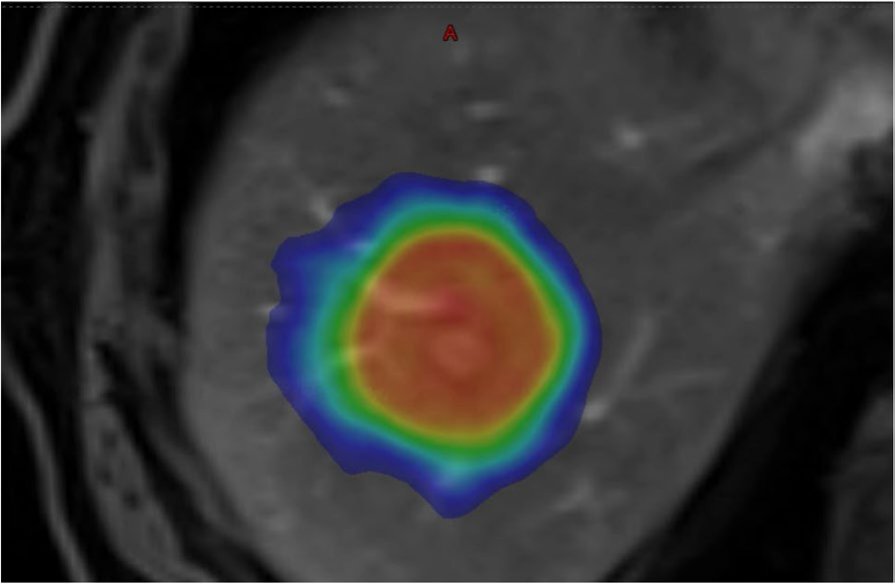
Planning MRI image of SBRT (24Gy / 3#) dose wash to a liver metastasis (image T1 weighted, fat saturated, post-liver specific contrast in the arterial phase)
